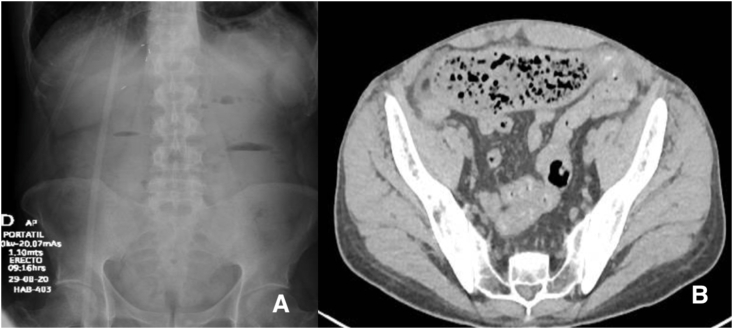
A) Abdominal x-ray. Air fluid levels in the small bowel, with no gas in the rectum. B) Abdominal computed tomography. Fecal content in the mid-jejunum.
